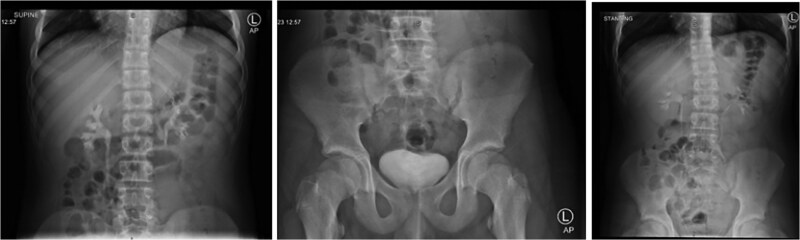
Abdomen/KUB X-ray: Revealed no evidence of intestinal obstruction or pneumoperitoneum in this study.
